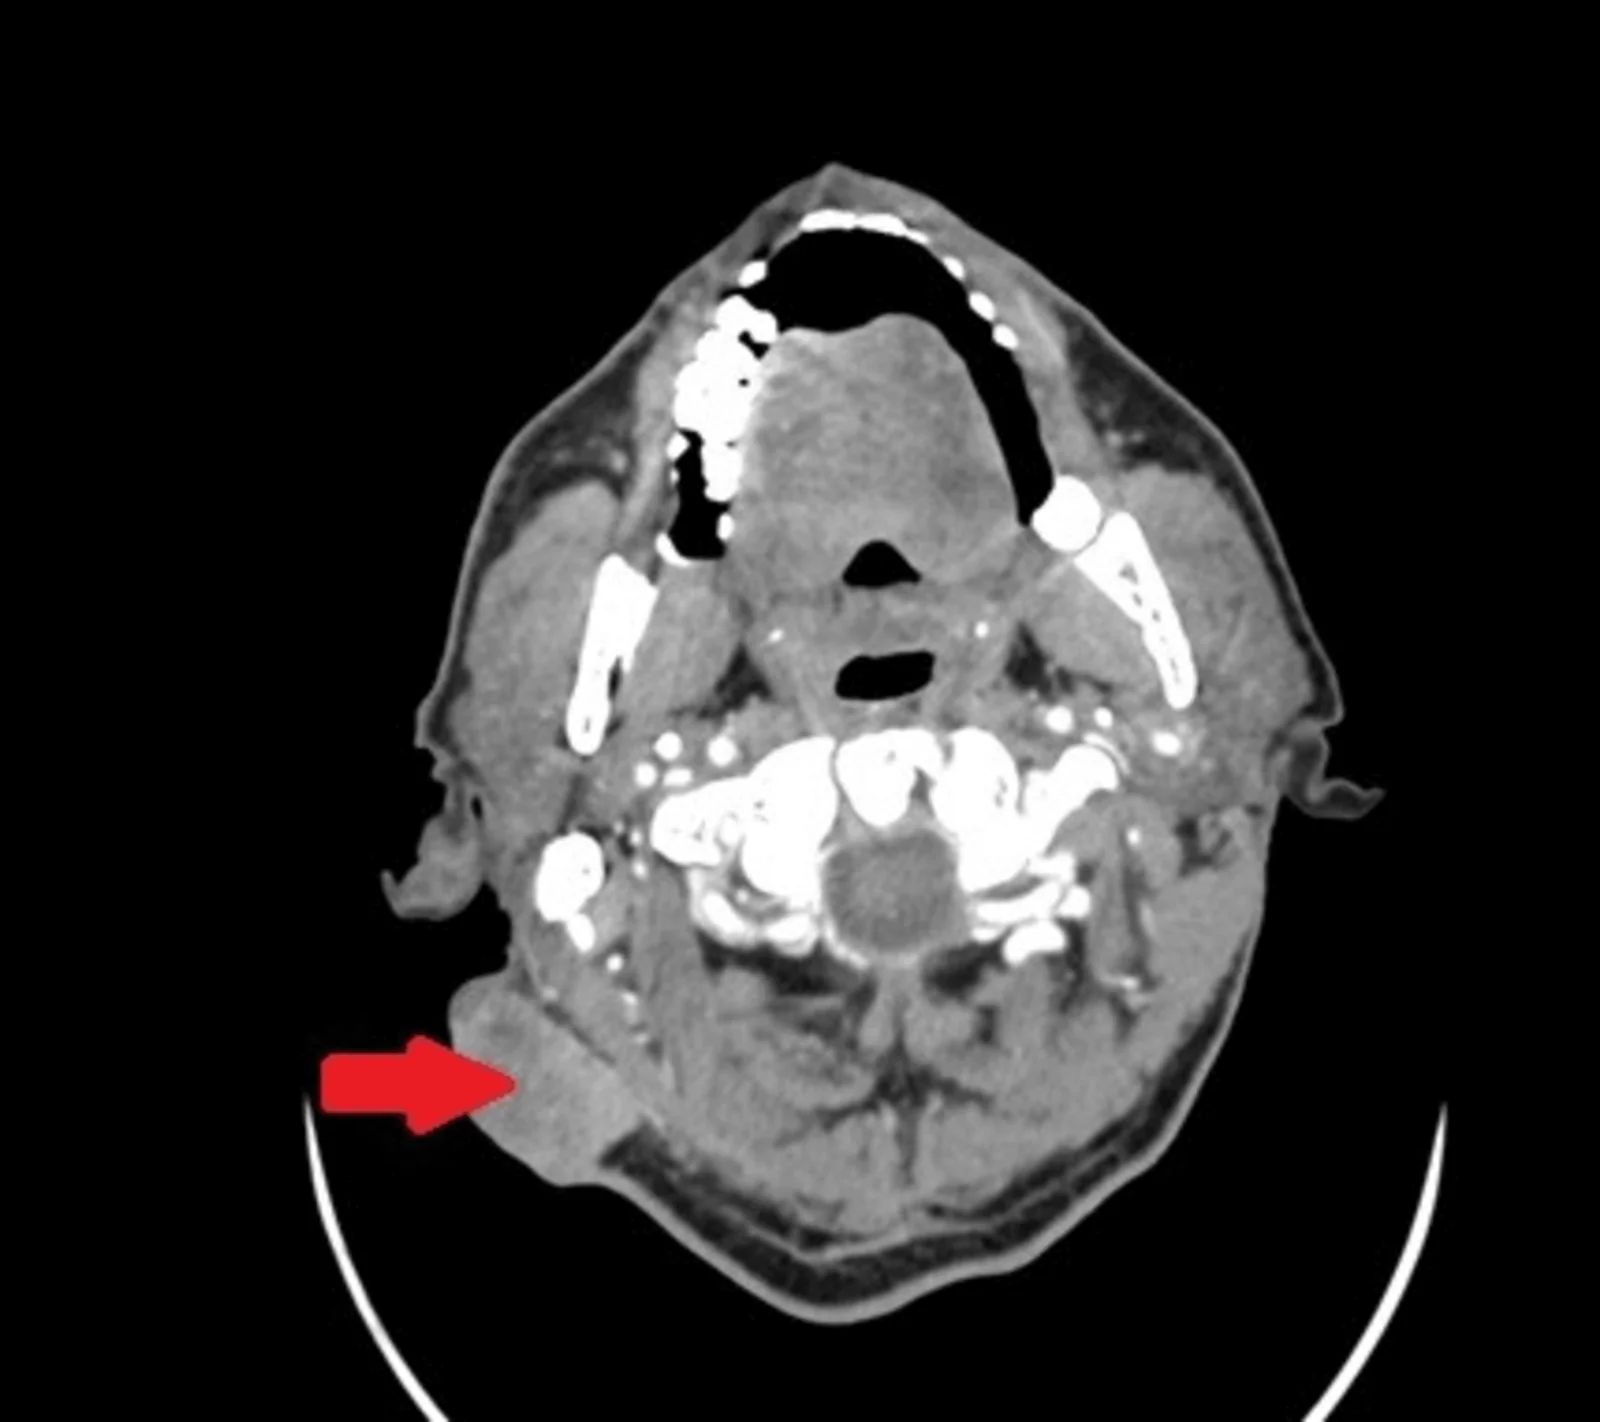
Contrast-enhanced CT scan demonstrating a well-defined, enhancing subcutaneous lesion in the right postauricular region (red arrow) without muscular or bony invasion.
